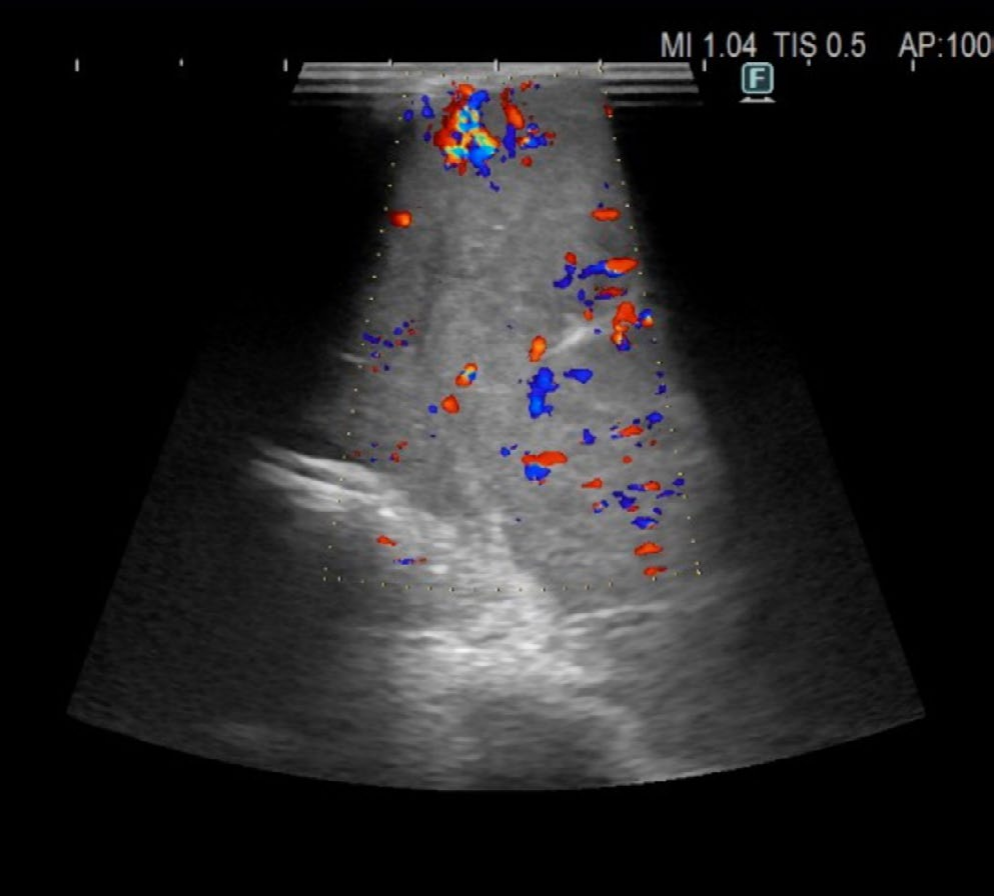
The ultrasound characteristics of mediastinal masses show: Increased vascularization in the intra‐ and perinodular color Doppler.
